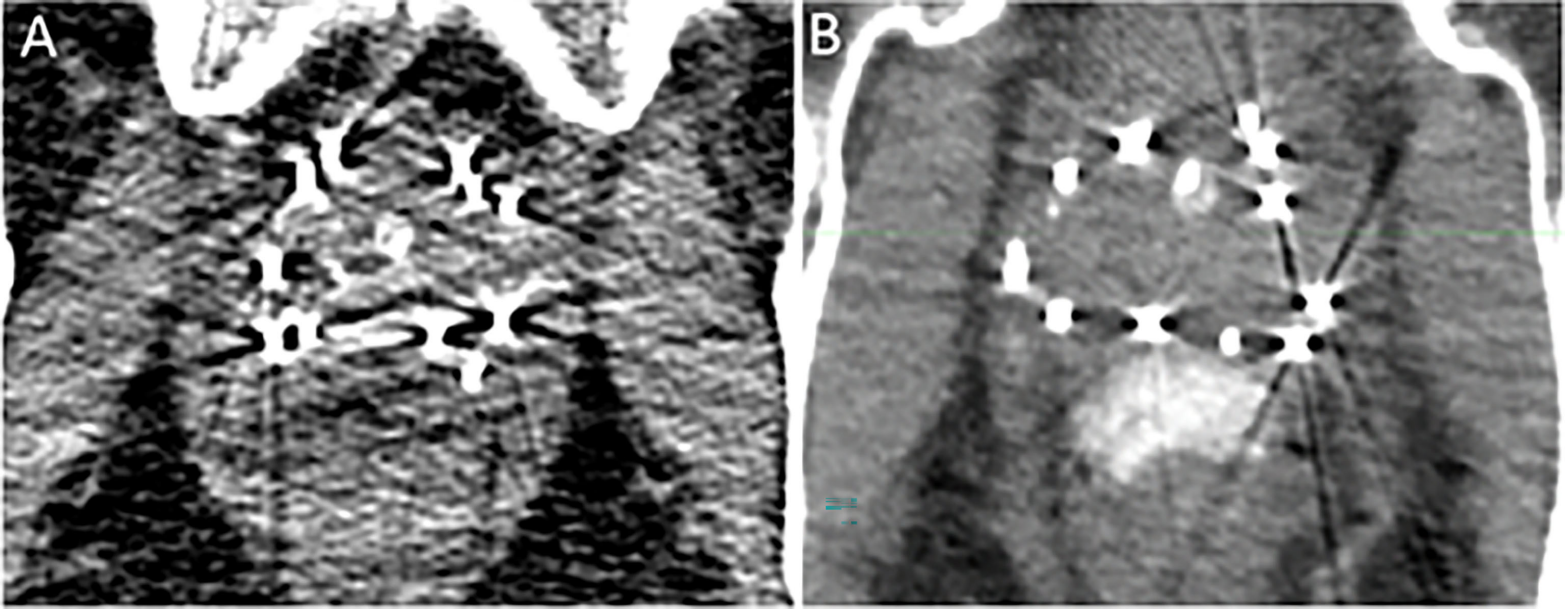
Day 0 non-contrast CT scan for postoperative dosimetry following low-dose-rate brachytherapy with placement of a standard SpaceOAR™ (A) and SpaceOAR Vue™ (B).
